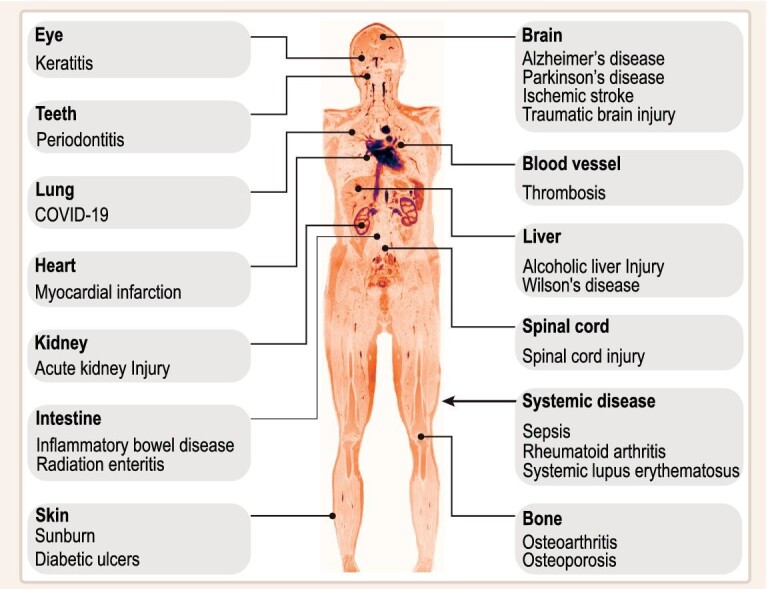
Representative applications of nanomaterials used in organ injuries. This pseudo-colour magnetic resonance image was obtained from a young Asian woman with permission.
